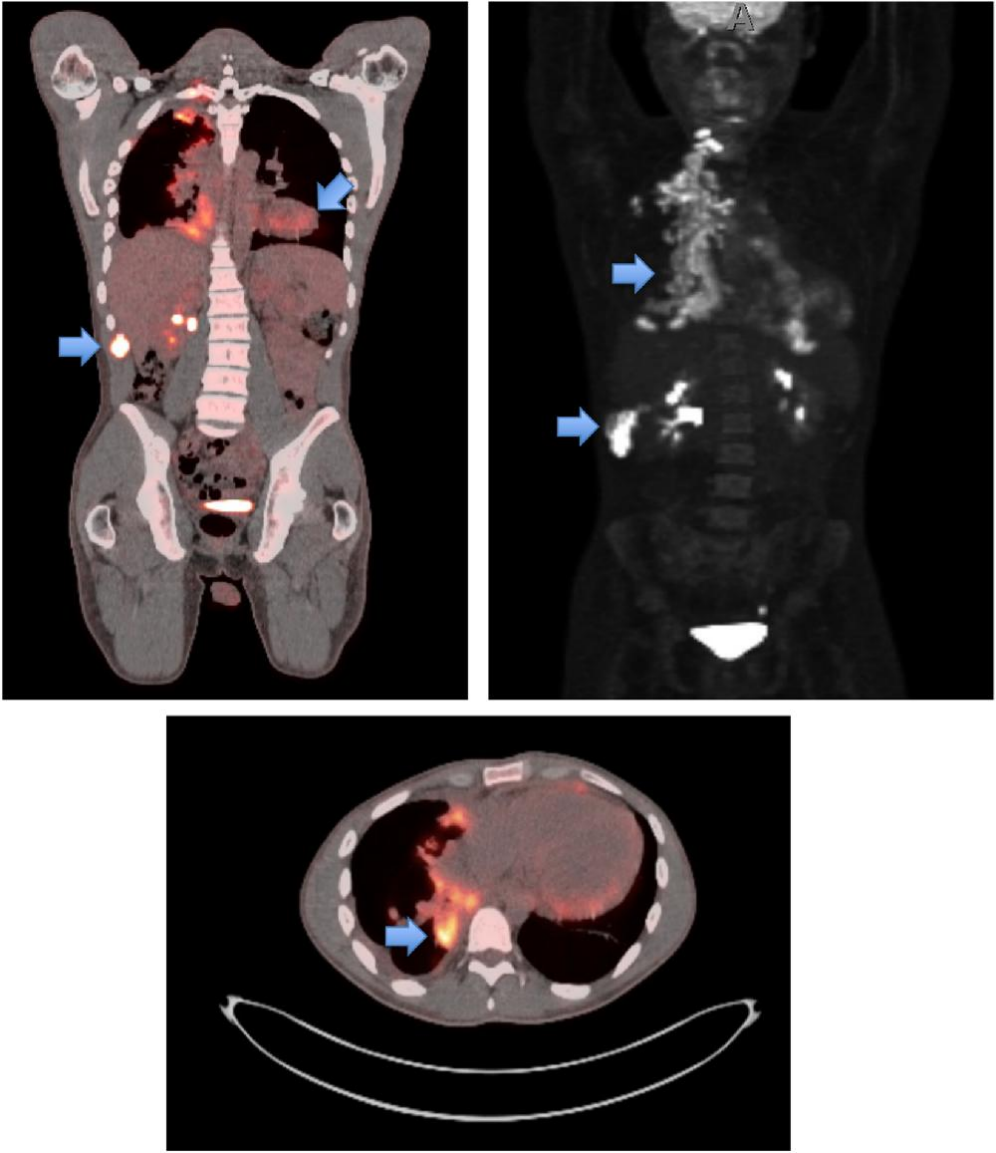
Whole-body PET/CT, demonstrating pulmonary, osseous, and cardiac involvement. Abbreviation: PET/CT, positron emission tomography/computed tomography.
